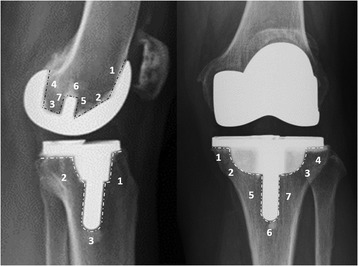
Postoperative radiographs for analysis of periprosthetic radiolucency. The tibial side was divided into 3 zones in the lateral view and 7 zones in the anteroposterior view (white dotted lines around the tibial component). The femoral side was divided into 7 zones on the lateral view (black dotted lines around the femoral component)
